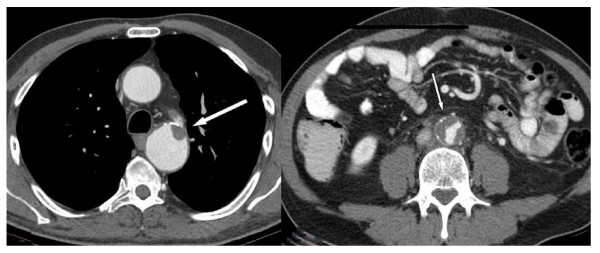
Contrast enhanced CT-scan after 2 cycles of chemotherapy. 2a: Chest CT: thrombotic deposit in the descending arch of thoracic aorta (arrow). 2b: abdominal CT: extensive endo-aortic thrombosis (arrow).
